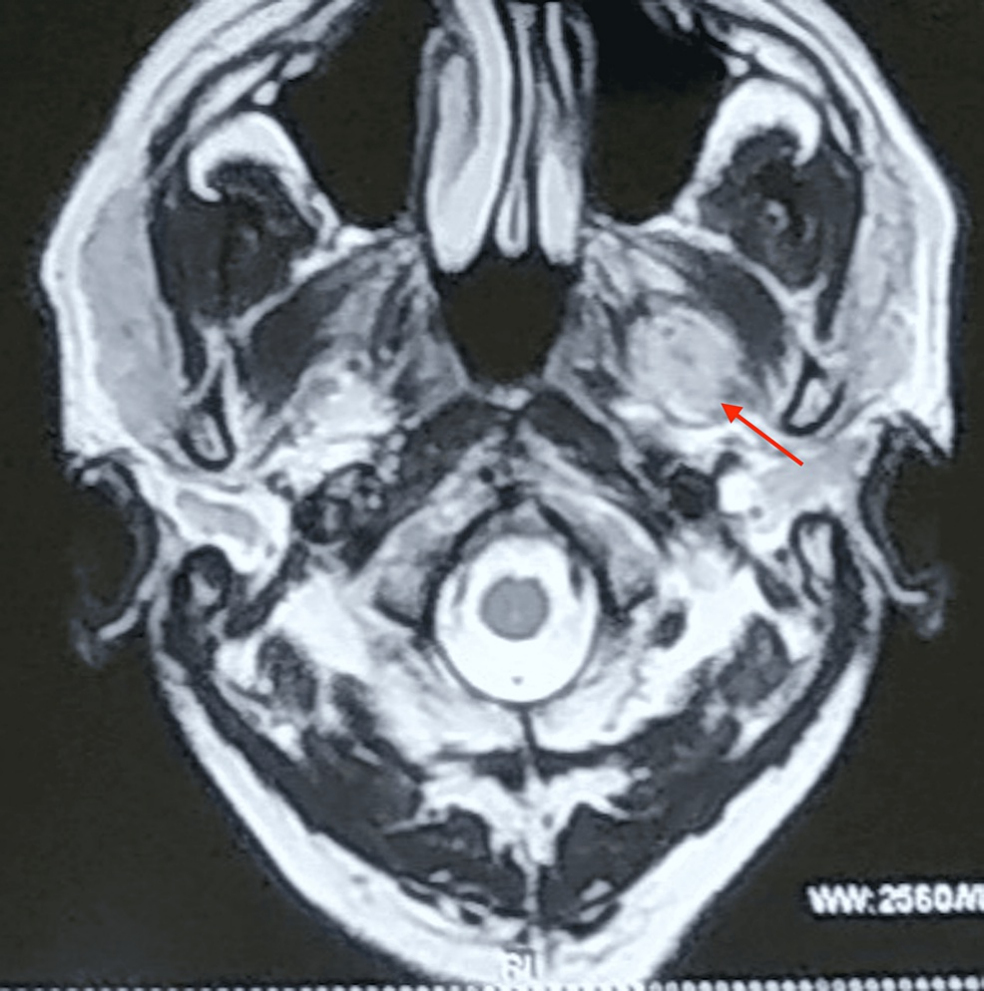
MRI T2 axial section shows hyperintense, peripherally enhanced collections in the deep intramuscular plane of the bilateral pterygoid fossa. MRI: magnetic resonance imaging
